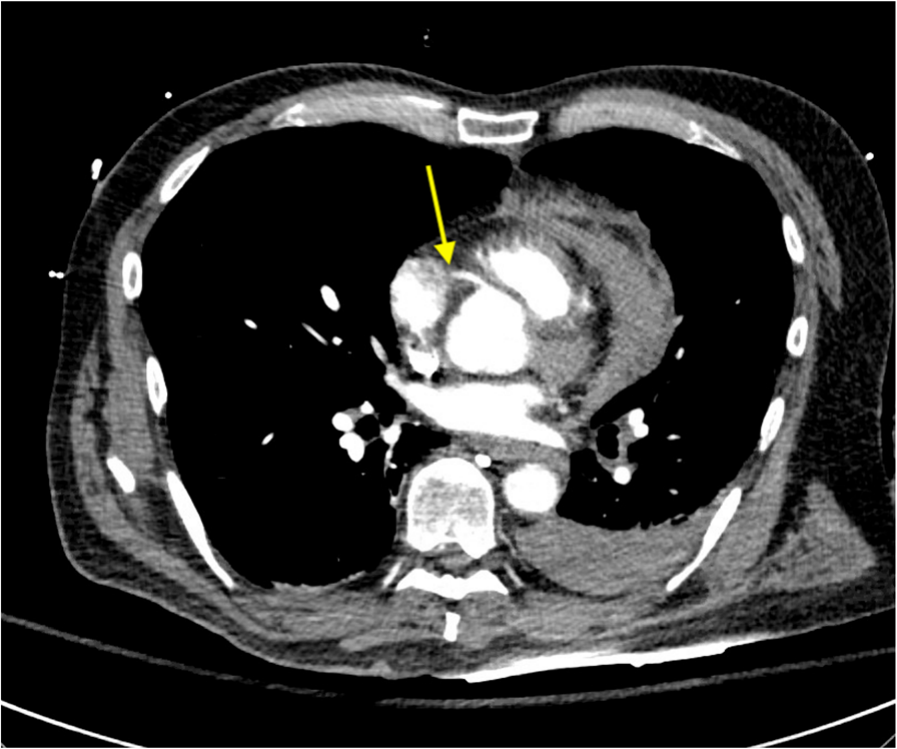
Axial view of Aortic CTA demonstrating occlusion of the proximal segment of the right coronary artery (yellow arrow)
